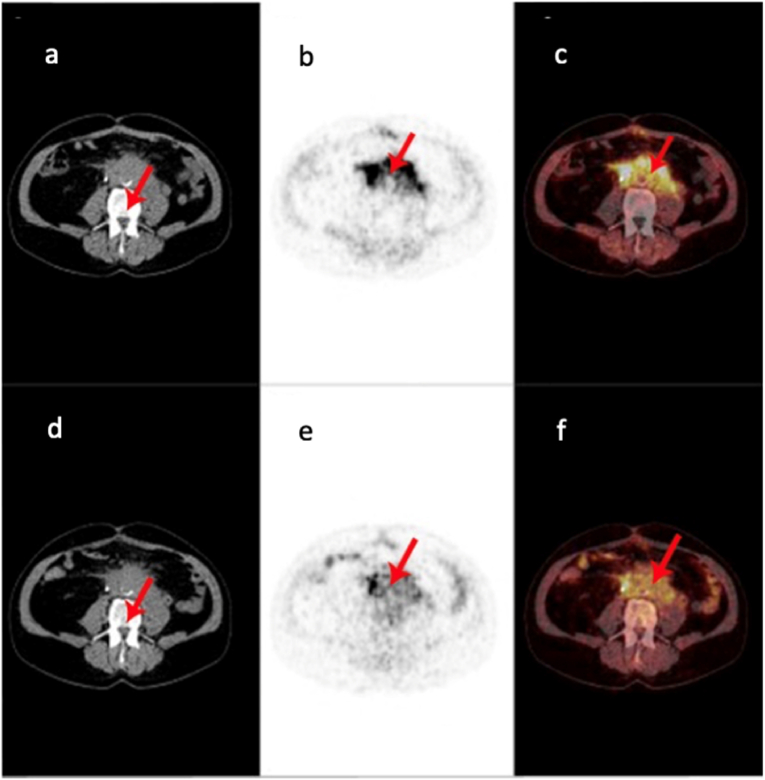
[68Ga]FAPI PET/CT (a–c) and 18FFDG PET/CT (d–f) images of restaging for urothelial carcinoma of the renal pelvis undergoing immunotherapy with pembrolizumab. Arrows indicate the retroperitoneal mass with high FAP-expression (upper row) and intense, inhomogenous FDG-avidity (lower row).
